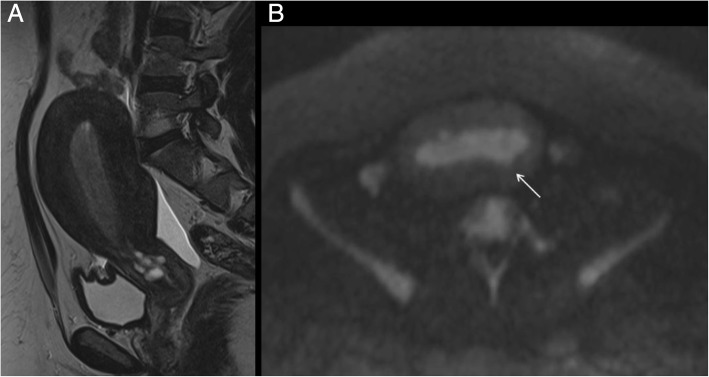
A 51-year-old woman with endometrioid adenocarcinoma invading < 50% of the myometrium. Sagittal T2-w MRI (a) shows an intermediate-signal intensity tumour with disruption of the low signal junctional zone but the extent of myometrial invasion is unclear. Corresponding axial DWI image (b) shows an area of high signal intensity within the endometrial tumour invading only the inner myometrium (arrow)
